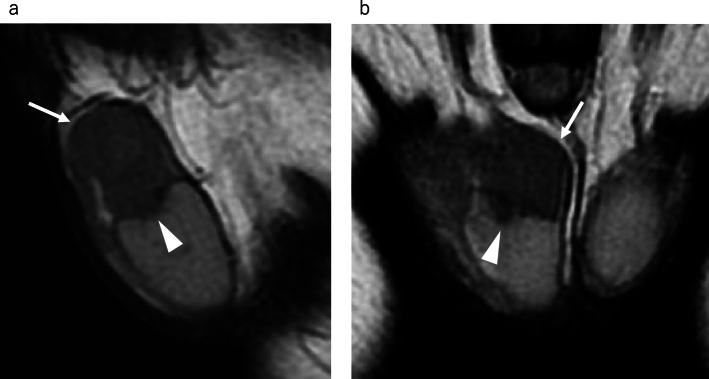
Seminoma in a 43-year-old male, extending into the extratesticular area and mimicking a benign extratesticular mass owing to minimal intratesticular component. a Sagittal T2-weighted MR image shows a homogeneous mass above the testis, exhibiting a mild hypointensity compared to the testis, mimicking extratesticular mass (white arrow). Mass is extending to the mediastinum of testis (white arrowhead). The figure is adapted from a study by Edo et al. [34]. b Coronal T2-weighted MR image shows a mass (white arrow) extending to the mediastinum of the testis (white arrowhead)
